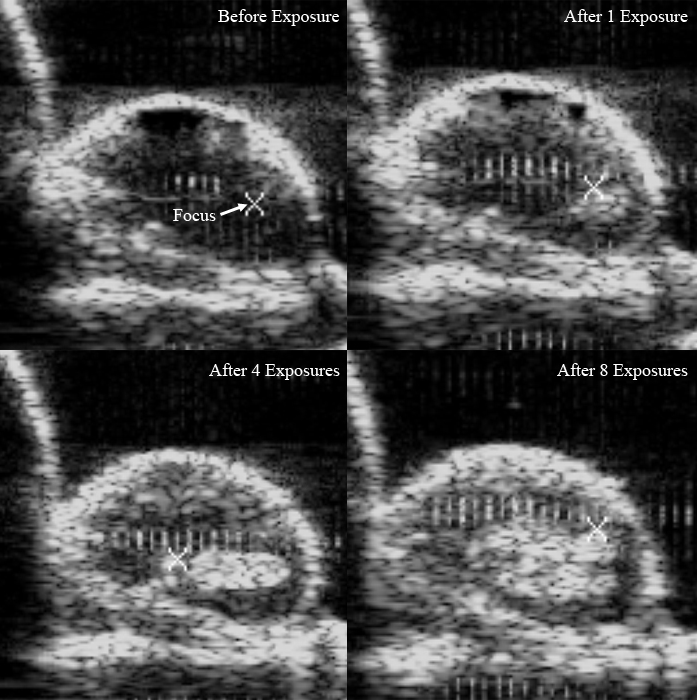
Ultrasound imaging of HIFU-induced cavitation. Time progression of a mechanical HIFU-exposure with B-mode ultrasound imaging. Bright hyperechoic spots generated by HIFU indicate regions of cavitation.
